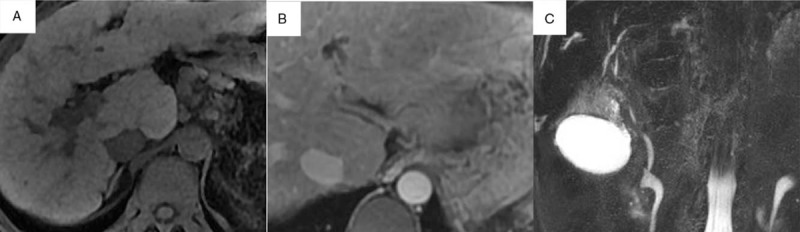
Imaging findings. A: MRI of the liver demonstrated liver cirrhosis. B: MRI of the liver showed mild dilatation in some intrahepatic bile ducts. C: Magnetic resonance cholangiopancreatography (MRCP) revealed low signal intensity in cystic ducts and obstructed bile ducts in the hepatic hilar region. MRI = magnetic resonance imaging.
